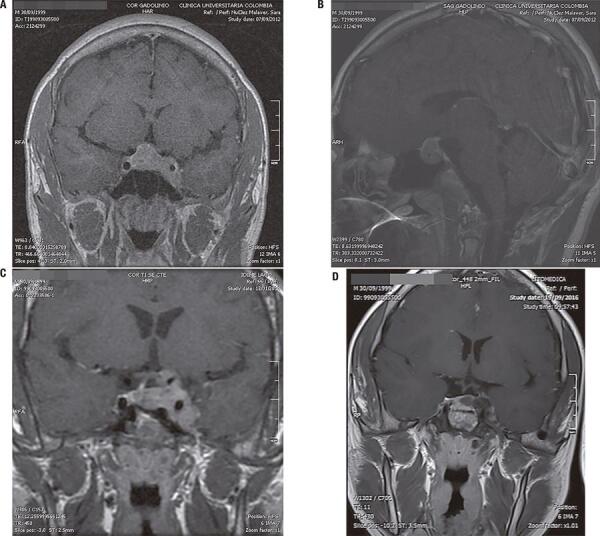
(A) Initial coronal T1-weighted magnetic resonance imaging (MRI). (B) Initial sagittal T1-weighted post-contrast MRI. (C) Coronal T1-weighted MRI 1 year after the second surgical procedure. (D) Coronal T1-weighted post-contrast MRI postcontrast 3 years after the second surgical procedure and 2 year after radioterapy plus medical treatment.
